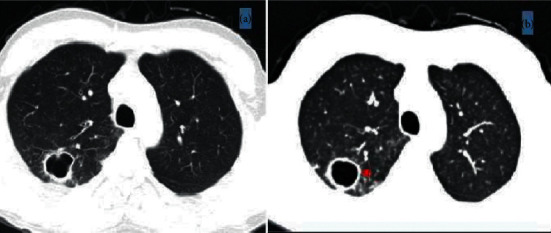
(a) Chest CT showing an apical pulmonary cavity in the right lung with bronchiolitis and hilar lymphadenopathy. (b) The chest CT performed 8 months later showing a regression of the pulmonary cavity (arrowhead).
